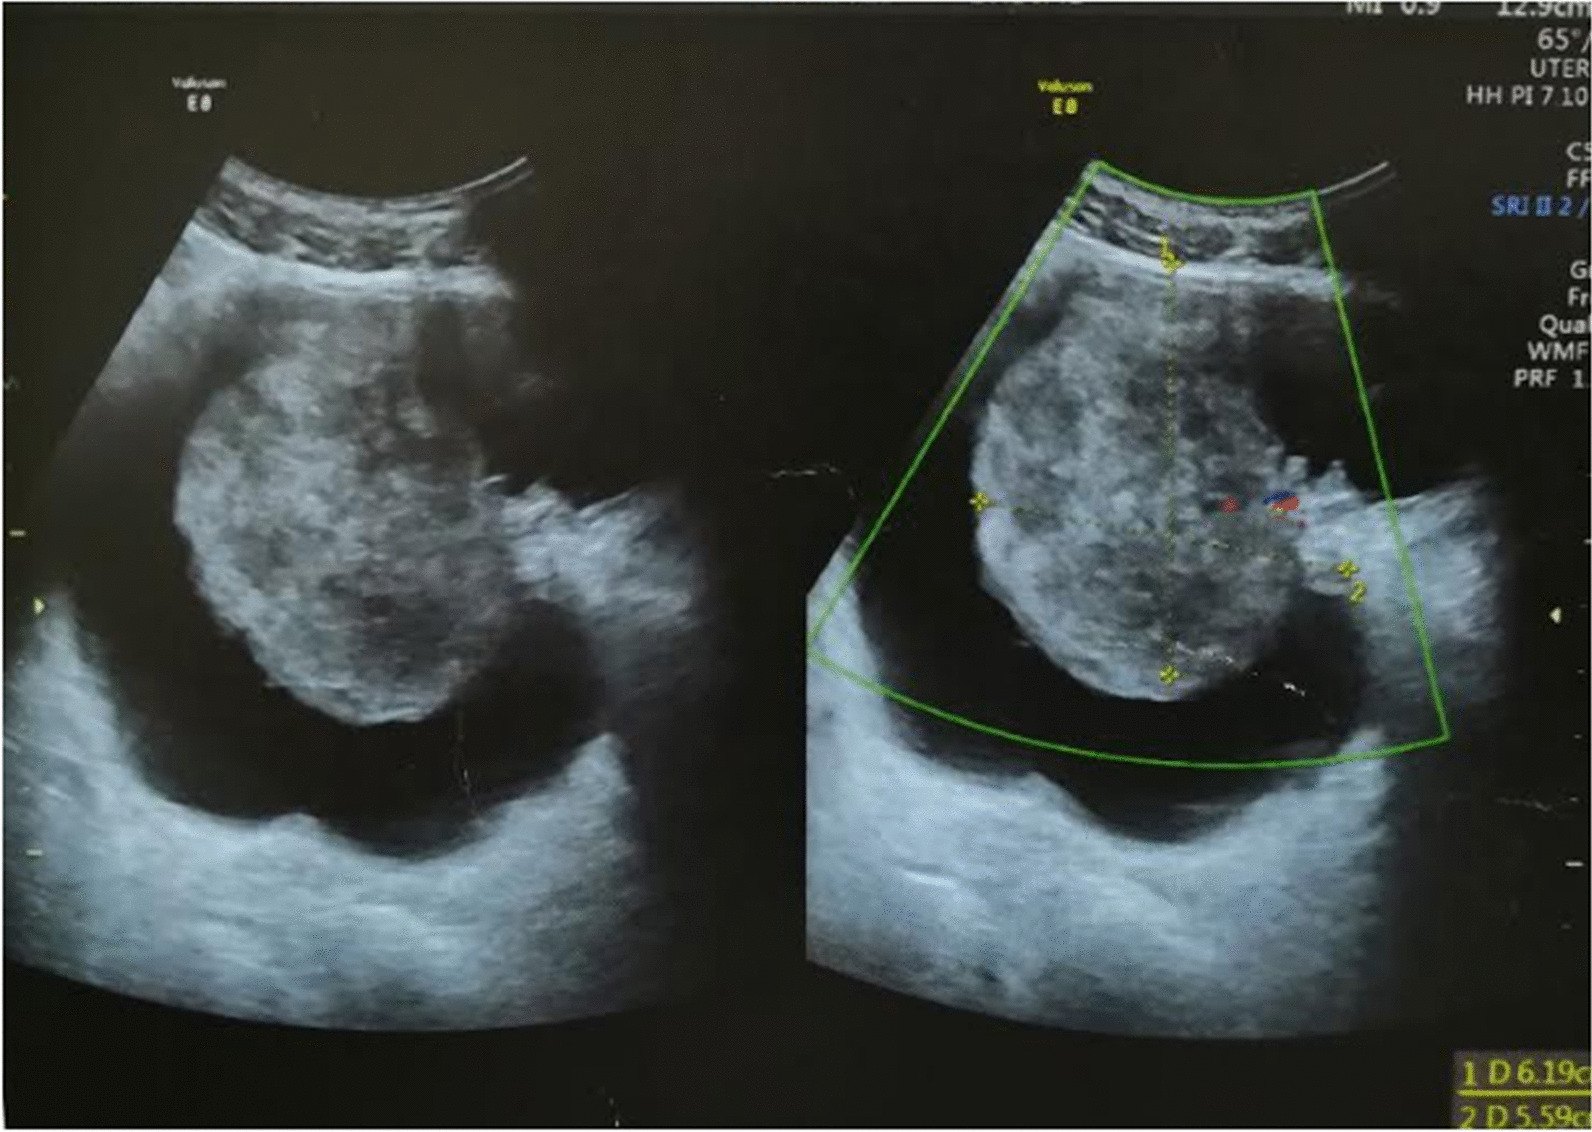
Pelvic ultrasound showed an echogenic mass of the left lateral wall of bladder
